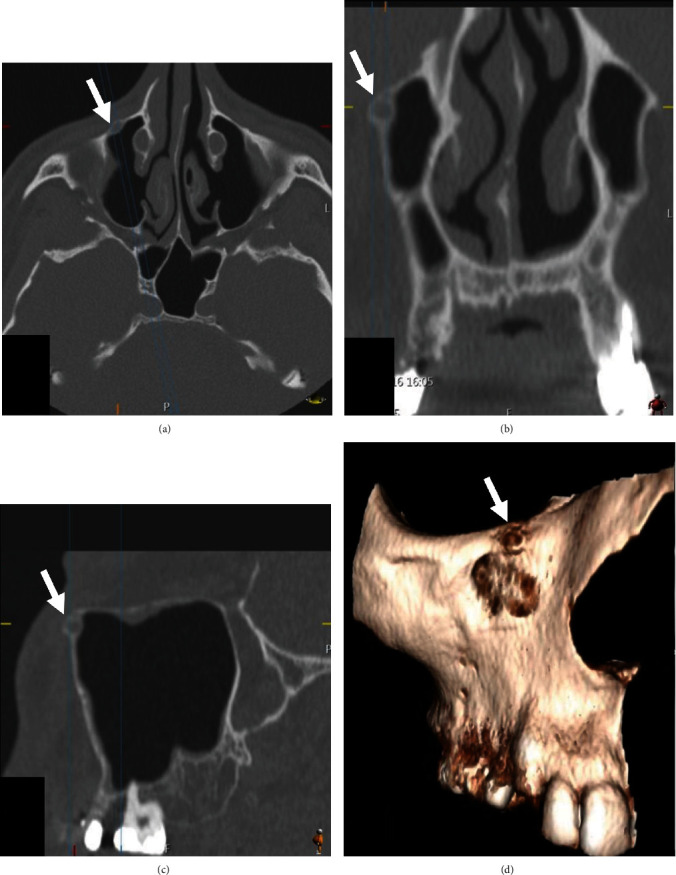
CT examination of the right maxilla in the bone window: (a) axial section, (b) corrected sagittal section, (c) coronal section, and (d) surface rendering; white arrows showing a small, well-defined, corticated, spherical, radiolucency in the superioanterior wall of the right maxillary sinus wall just below the infraorbital rim and medial to the infraorbital foramen.
